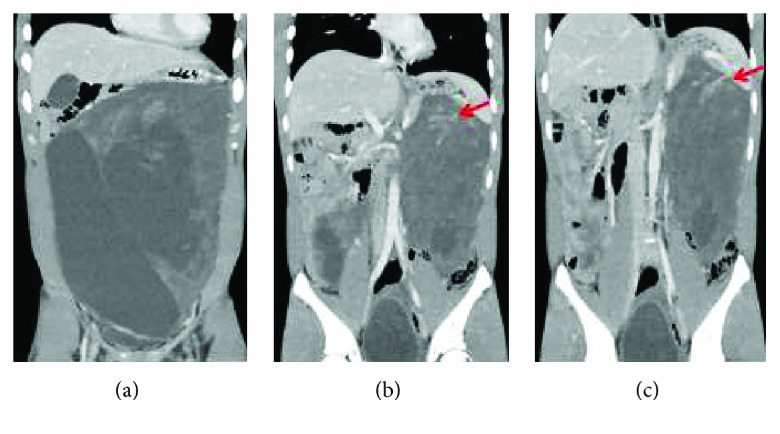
(a–c) CT scan with intravenous contrast (coronal view): the vessel seen from the left gastroepiploic artery to the tumor (red arrow).
